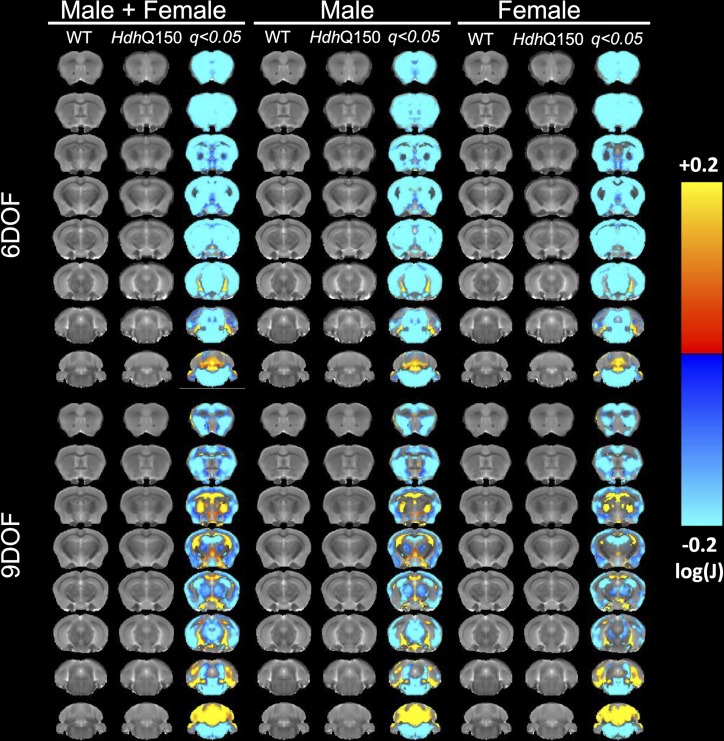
TBM on high resolution ex vivo T_2_-weighted MR images. To reduce potential partial volume effects in sub-regional comparisions *ex vivo* high resolution MR images were acquired to achieve a spatial resolution 2.5 higher than *in vivo*, as well as a >2x higher SNR, while avoiding motion related artefacts. This afforded a more precise investigation of subtle effects that might not be evident on *in vivo* images. A more defined sub-regional pattern of statstical differences between WT and *Hdh*Q150 was evidence using this approach. Color scales indicate statstically significant volume decreases (cold colors) and increases (warm colors) with correction for multiple comparisons (FDR with q<0.05).
